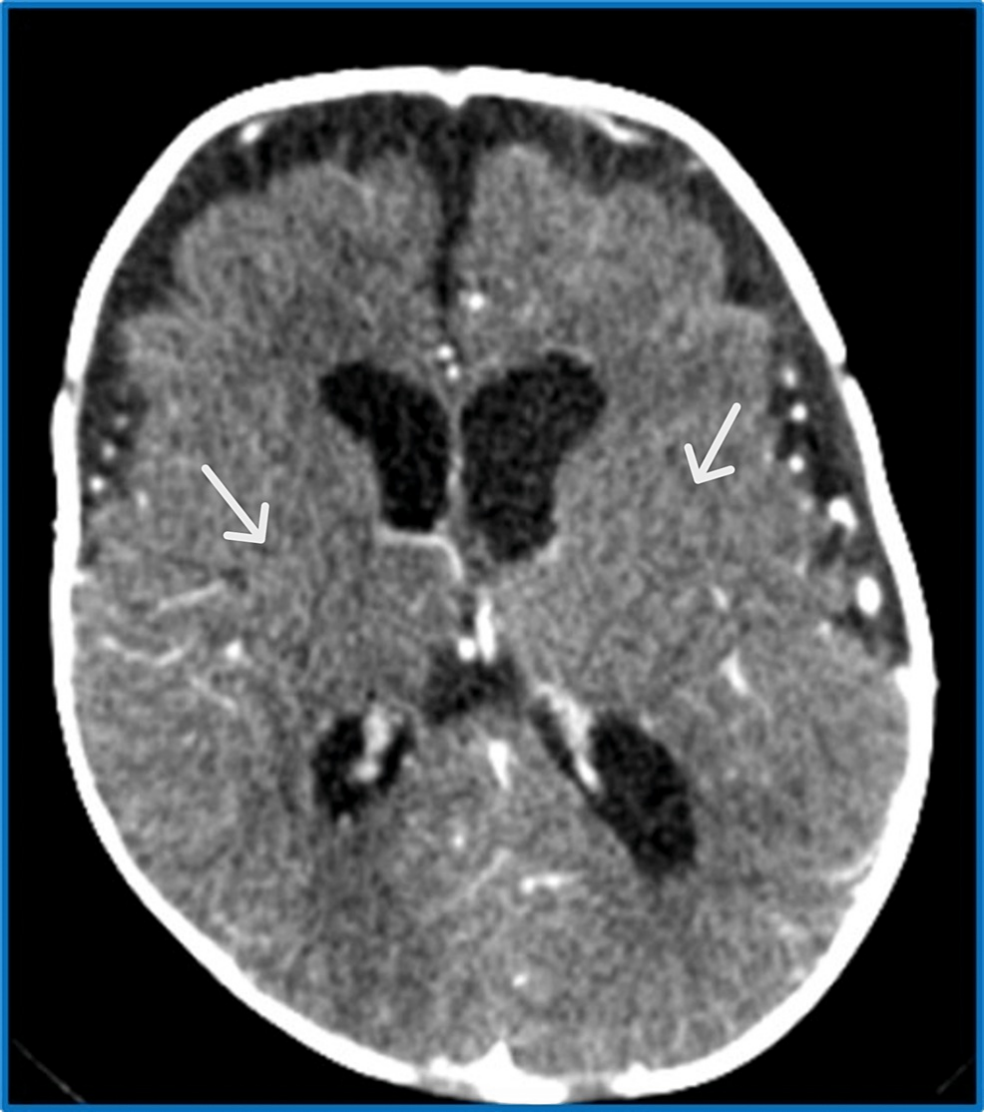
Brain CT on the day of admission showed signs of increased intracranial pressure (loss of gray matter and white matter differentiation marked by white arrows)
